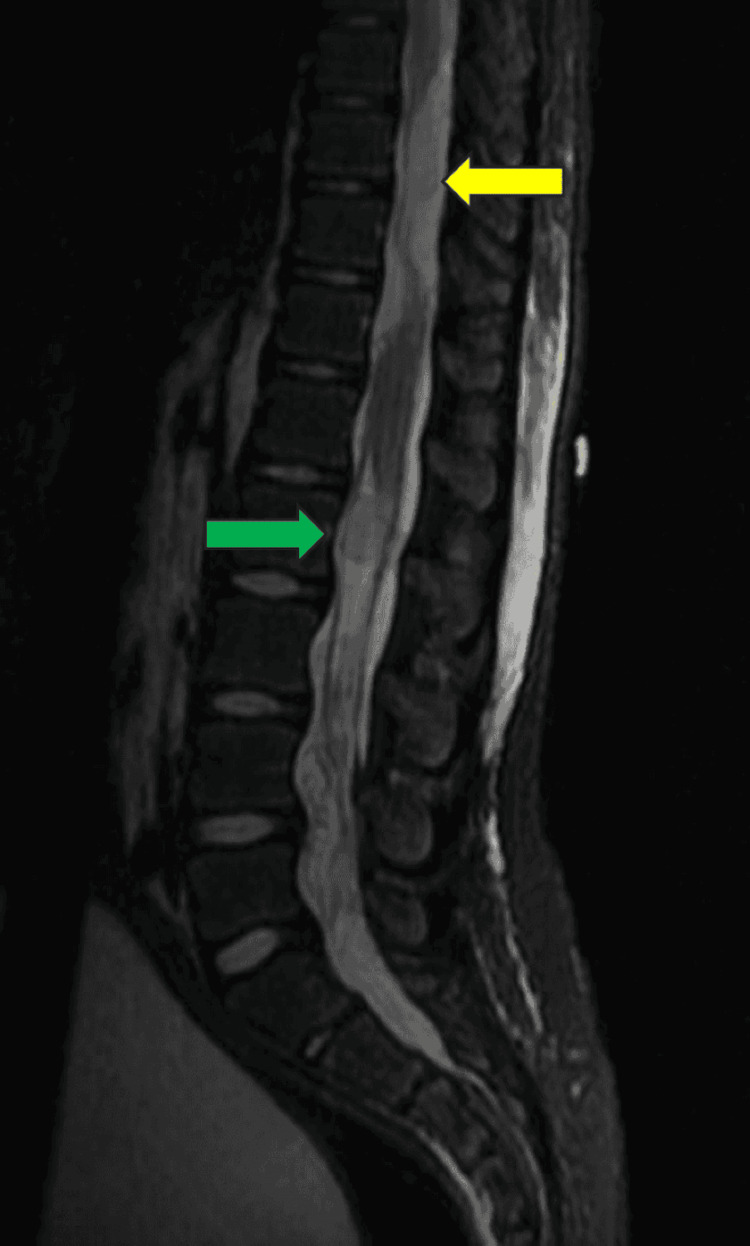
Sagittal T2 MRI with the yellow arrow pointing to the hyperintense extramedullary tumor involvement of the thoracic and lumbar spine and a green arrow pointing to intramedullary involvement of the lumbar spine with metastatic disease. The spinal cord is hypointense in this image.
